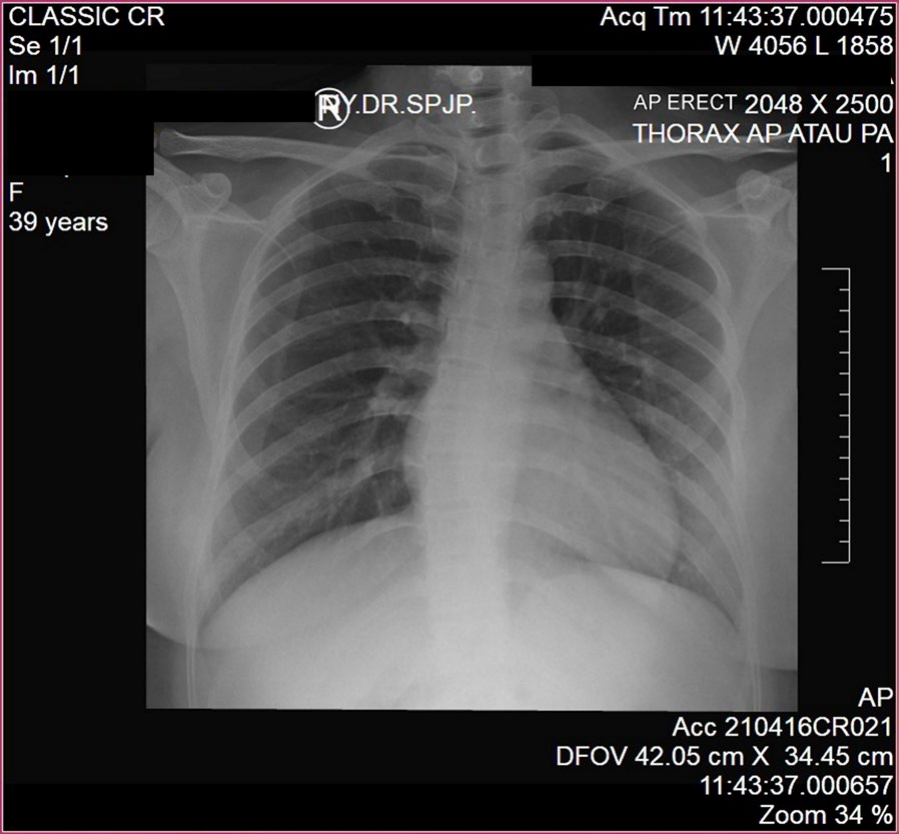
Chest X-ray showing normal radiological finding
